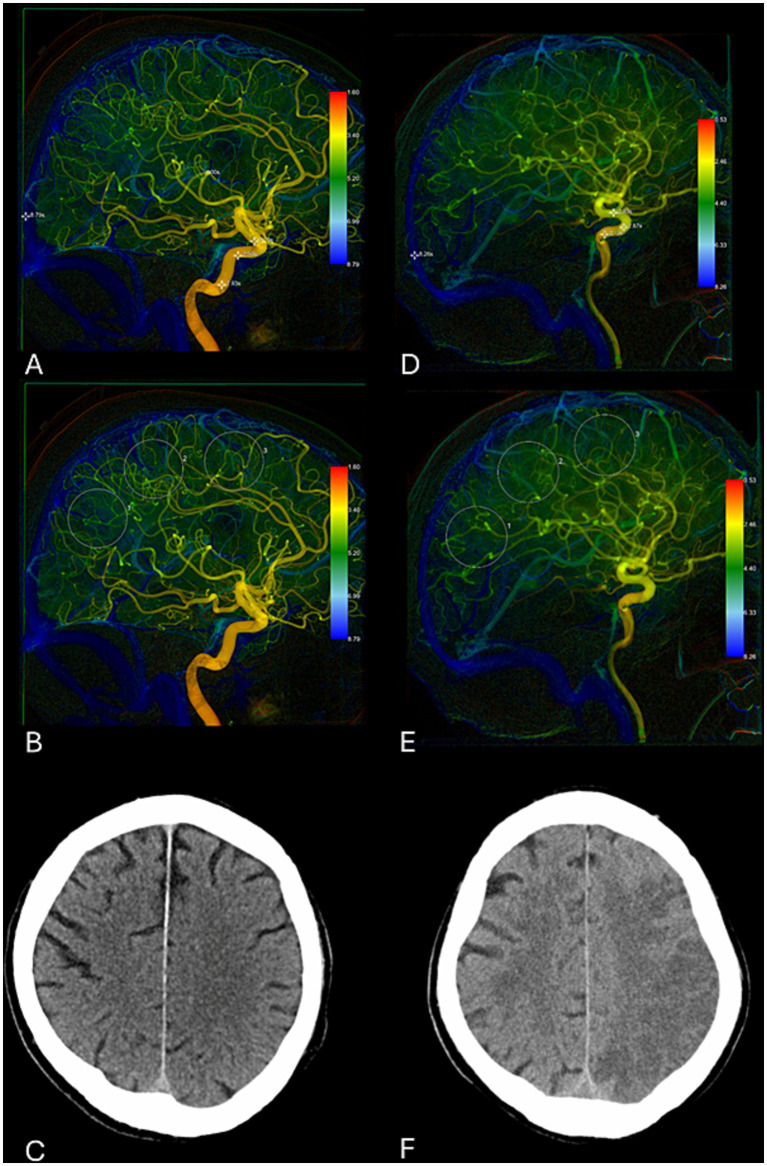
(A,B) Color-coded image from a lateral 2D DSA series of a 63-year-old patient shows arterial POI placement at the carotid artery and venous POI placement at the superior sagittal sinus, positioned 2 cm anterior to the confluence of sinuses. In this patient, who was treated with TNK and developed minor infarction mTT was calculated to be 2.66 s. (C) Non-contrast CT of the same patient within 24 h after thrombectomy without significant infarction. (D,E) A color-coded image from a lateral 2D DSA series of a 72-year-old patient treated with rtPA. Analogous to figure POIs and ROIs were placed. MTT was calculated to be 3.72 s and the patient subsequently developed large infarction. (F) Non-contrast CT showing extensive infarction involving the left hemisphere.
